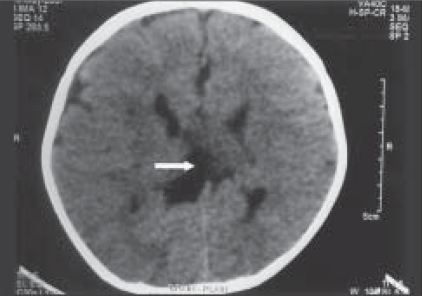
Axial CT brain showing parallelism of ventricles with a small inters hemispheric cyst (white arrow) and no impression of splenium suggestive of hypogenesis of corpus callosum.
